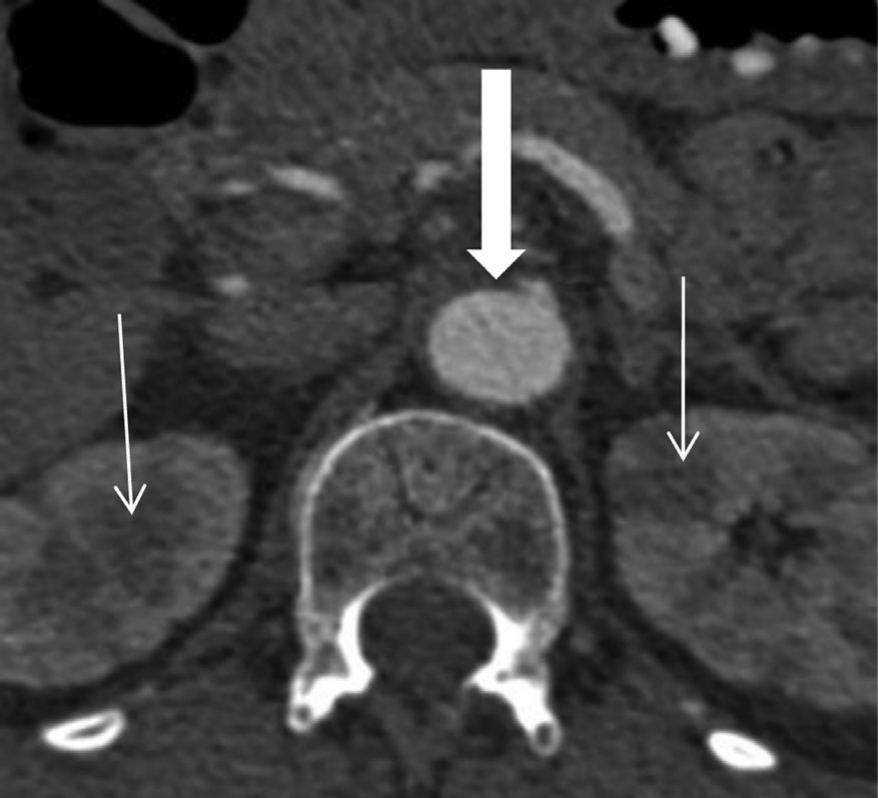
Axial CT Abdomen demonstrating renal infarcts and mural thickening of the aorta at the level of the celiac axis (block arrow).
